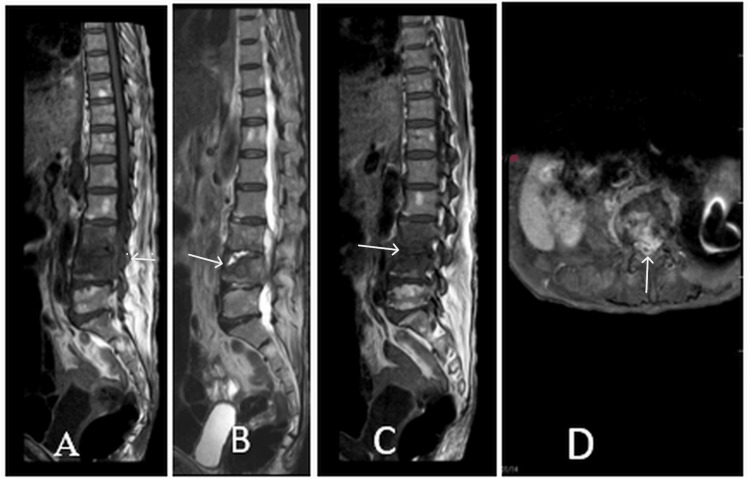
MRI of the lumbosacral spine (Case 4) (A) Sagittal T1-weighted image: hypointense marrow signal changes at L4 and L5 vertebral bodies (arrows). (B) Sagittal T2-weighted image: hyperintense collections within the L4–L5 intervertebral disc space (arrows). (C) Bone window image: vertebral endplate destruction and disc space narrowing (arrow). (D) Axial image: mild spinal canal compromise at the affected level (arrows). Findings are consistent with infective spondylodiscitis at L4–L5.
